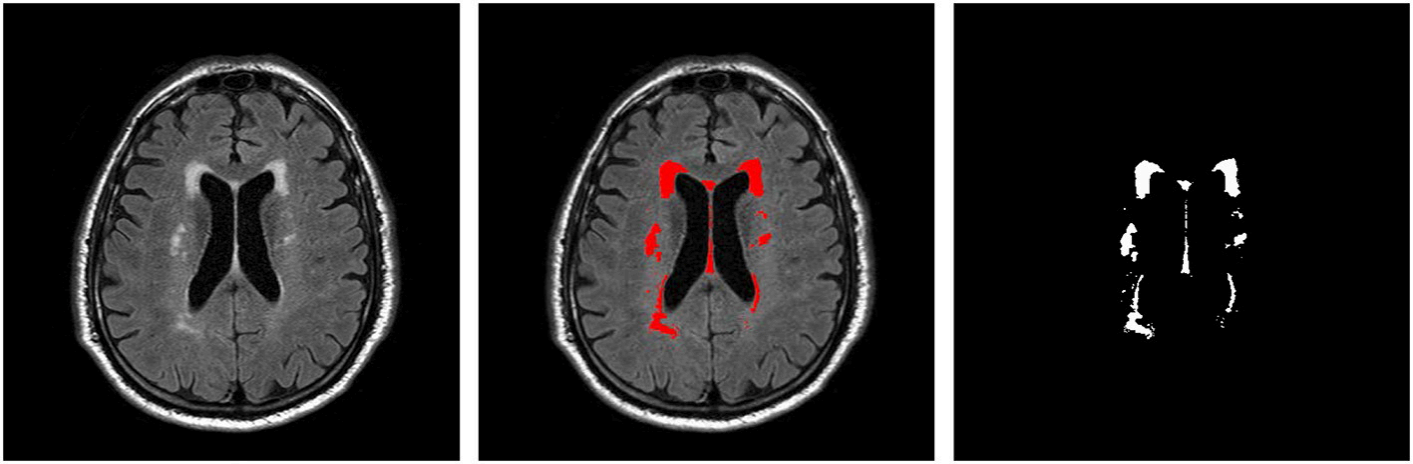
An example T_2_ FLAIR image from one subject showing multiple white-matter hyperintensity (WMH) lesions; the results of WMH segmentation using the lesion prediction algorithm (LPA) showing in red, and a WMH binary mask after tissue segmentation, which was used in shape feature extraction.
